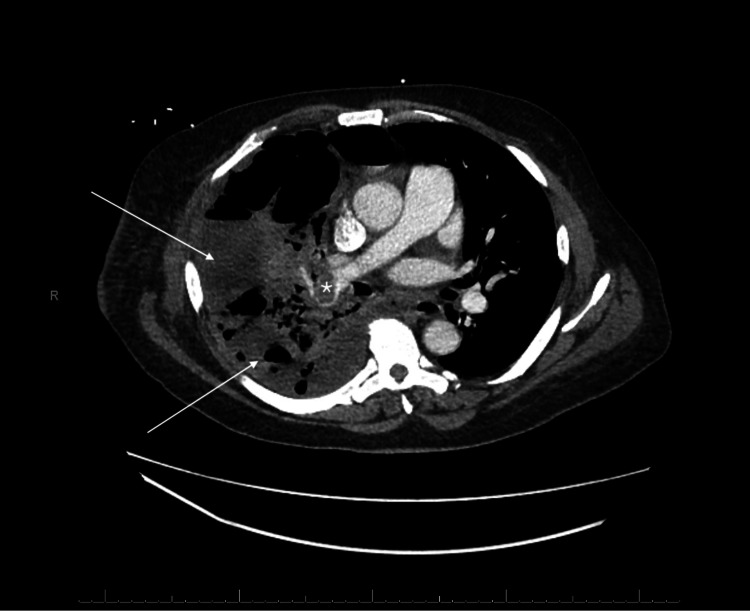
Chest CT with intravenous contrast showing acute pulmonary embolism in the right pulmonary artery (star) with extensive areas of necrotizing pneumonia and associated empyema (arrows)
